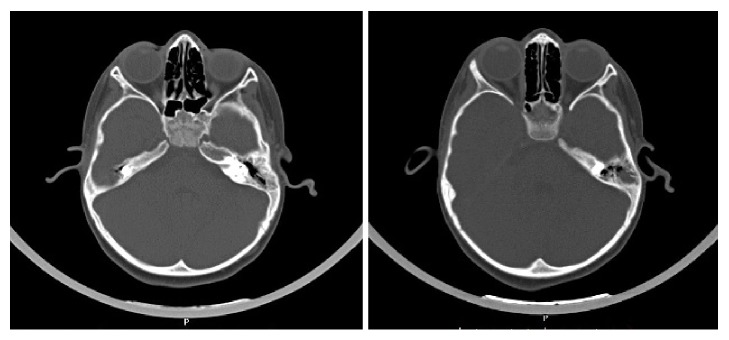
Postchemotherapy CT scans of the patient.
